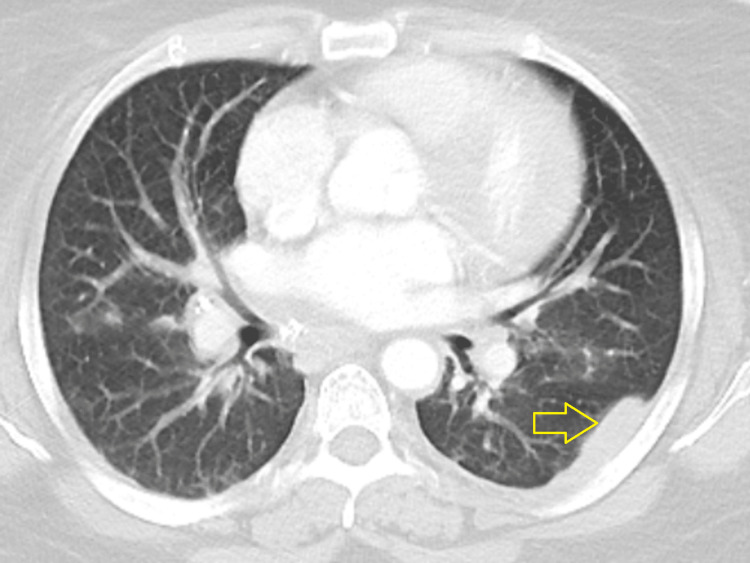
CT chest with IV contrast showing left pleural-based lesion (yellow arrow) along the superior segment of the left lower lobe.
